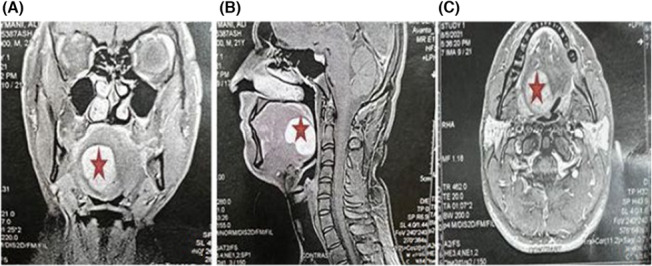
Soft tissue mass with irregular and smooth border in the right posterior aspect of tongue was seen on MRI sections. (A) Coronal view, (B) Sagittal view, (C) Axial view.
